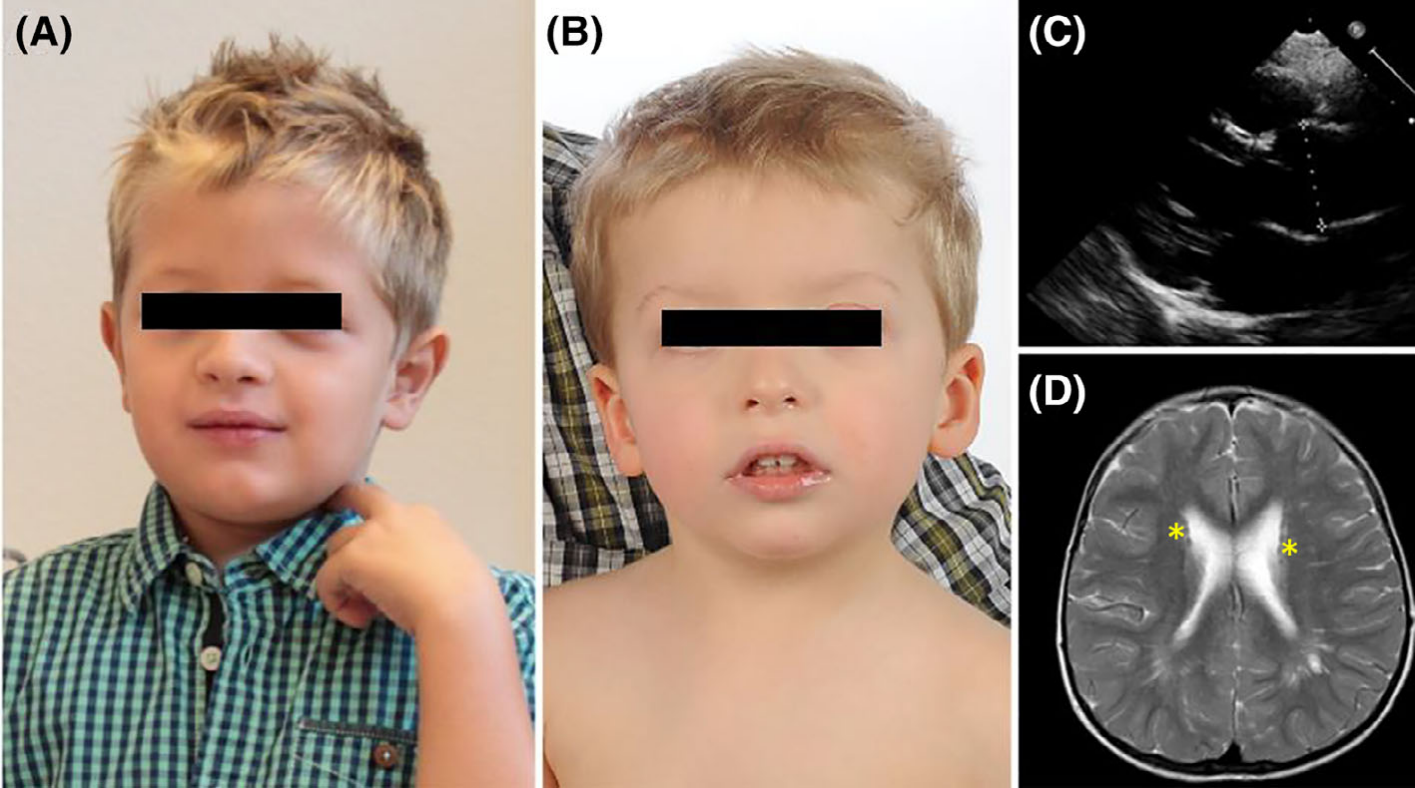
Clinical presentation of mannosyl‐oligosaccharide alpha‐1,2‐mannnosidase (MAN1B1)‐congenital disorders of glycosylation (CDG). A, Picture of patient 1 (P1). B, Picture of patient 2 (P2). C, Echocardiography of P1 in the parasternal longitudinal axis shows a slight extension of the aortic bulbous (28 mm; 28 mm/m^2^ body surface area; reference value 15‐20 mm/m^2^ body surface area). D, Neurological findings of subject P 2: A magnetic resonance image (MRI) in T2. The image revealed multiple subependymal heterotopia of the gray matter, exemplarily marked with yellow stars
